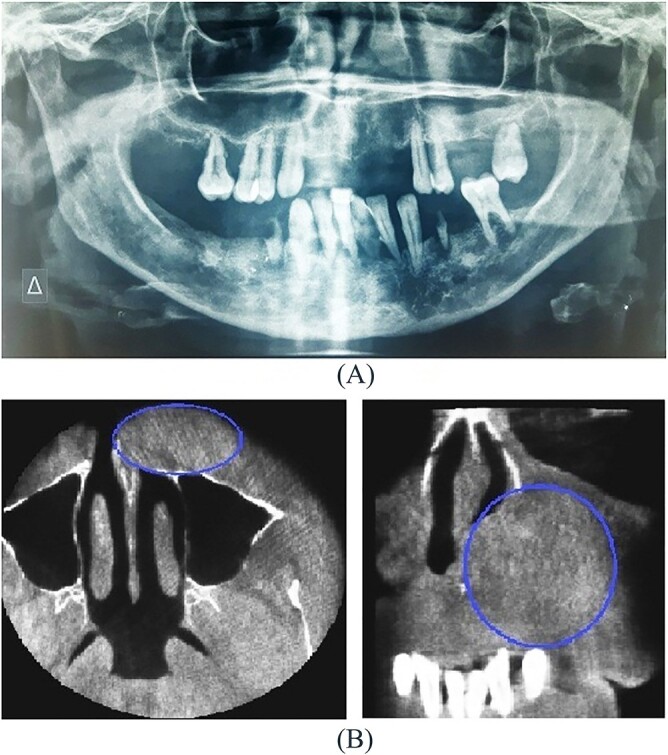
(A) Orthopantomogram, (B) CBCT imaging of soft tissue mass.
